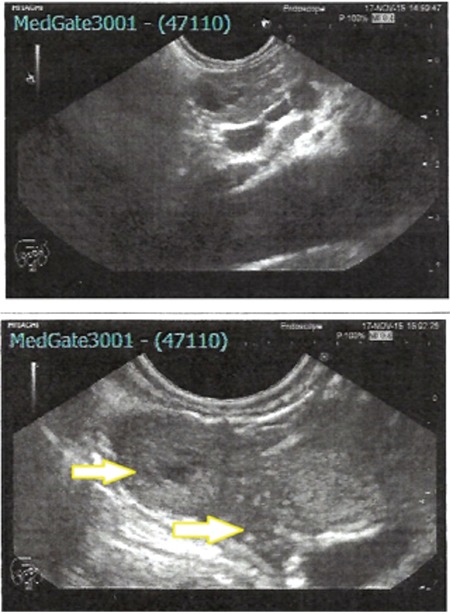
Endoscopic ultrasound images of the index patient showing the two pancreatic lesions (yellow arrows)
